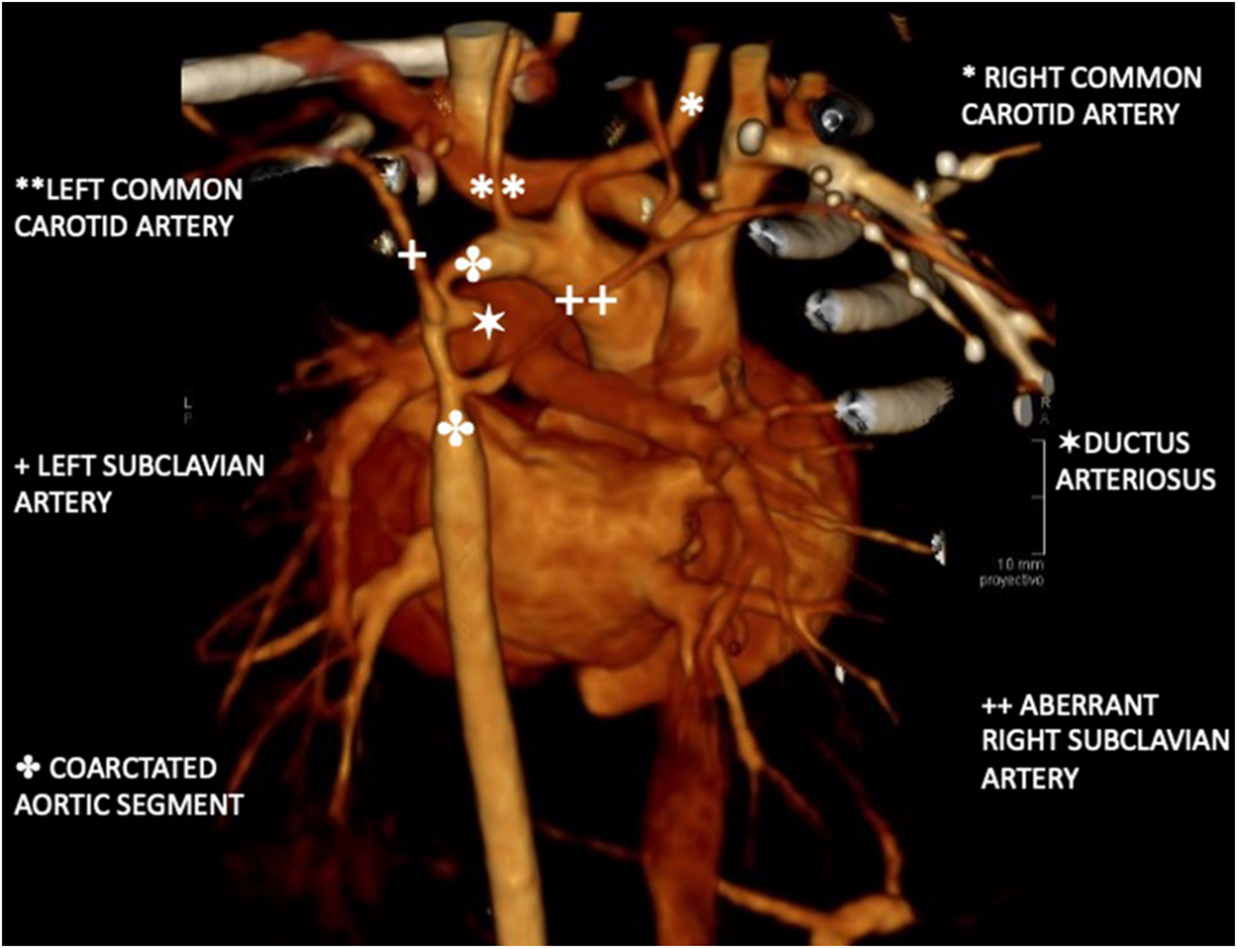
Three-dimensional reconstruction of aortic CT angiography. Periductal aortic stenosis extends 10 mm craniocaudally with a 1 mm diameter. Distal aorta measures 4 mm, widening to 5 mm at the diaphragmatic hiatus. An aberrant right subclavian artery collapses the esophagus. The left common carotid artery is reduced to 2 mm. at its origin. A partially filled ductus is observed with a diameter of 3 mm.
